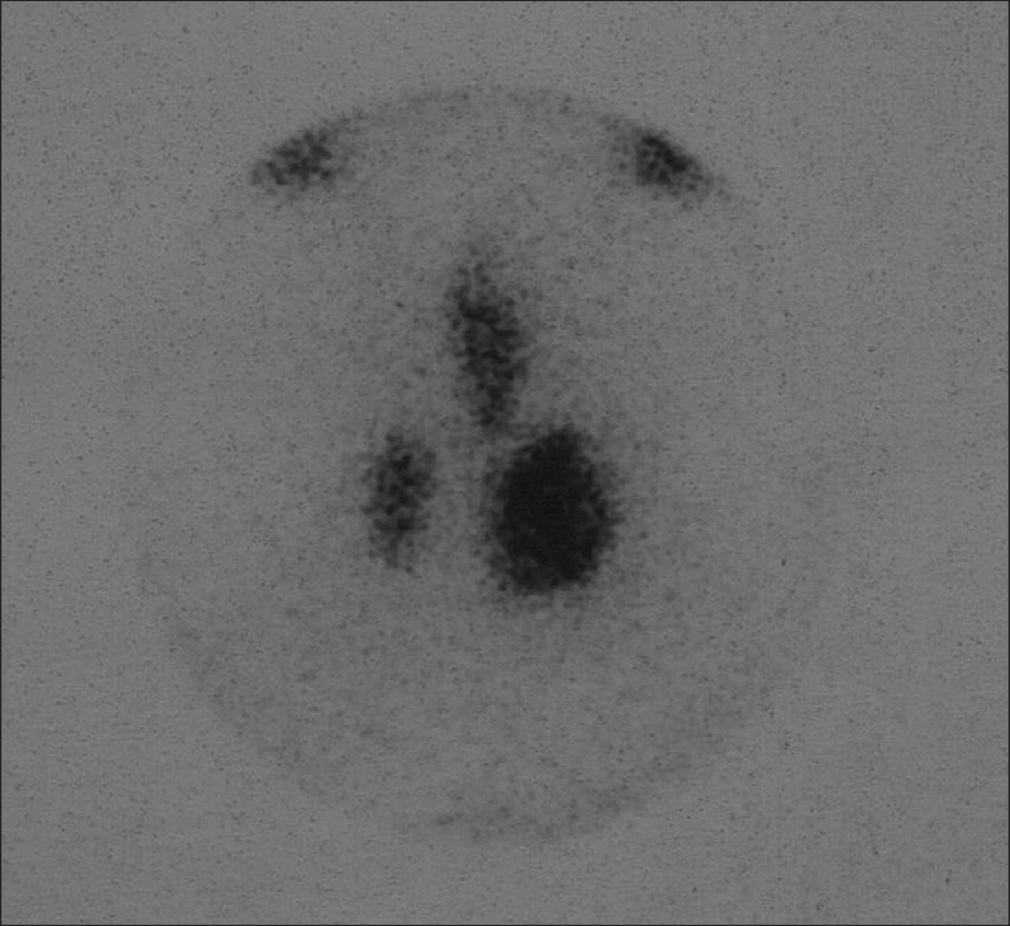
Tc-99m thyroid scintigraphy (before the completion thyroidectomy). There exists 3 distinct activity foci compatible with residual thyroid tissues in the right thyroid lobe, left thyroid lobe and pyramidal lobe
